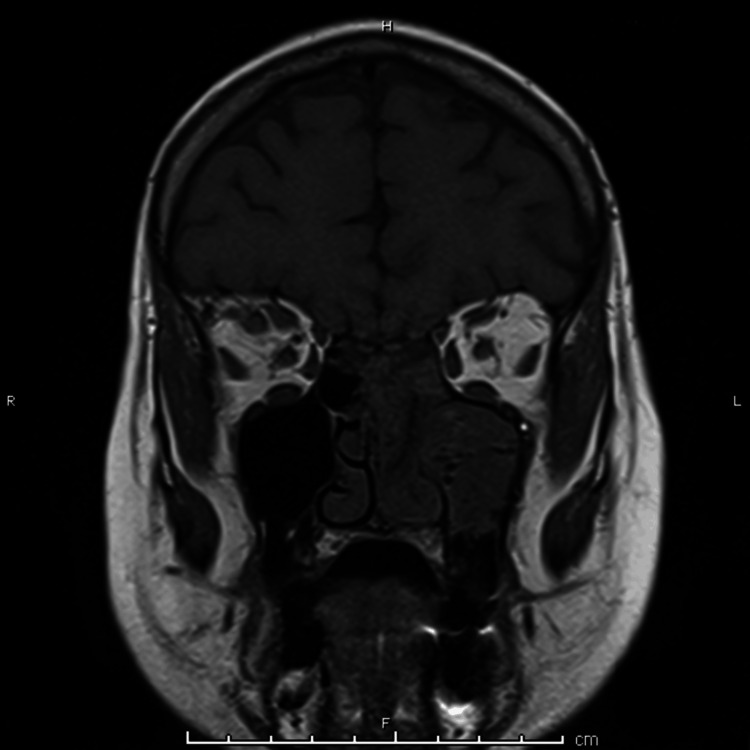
Coronal view of MRI orbits with contrast demonstrating sphenoid sinus opacification and right-sided orbital fat stranding
